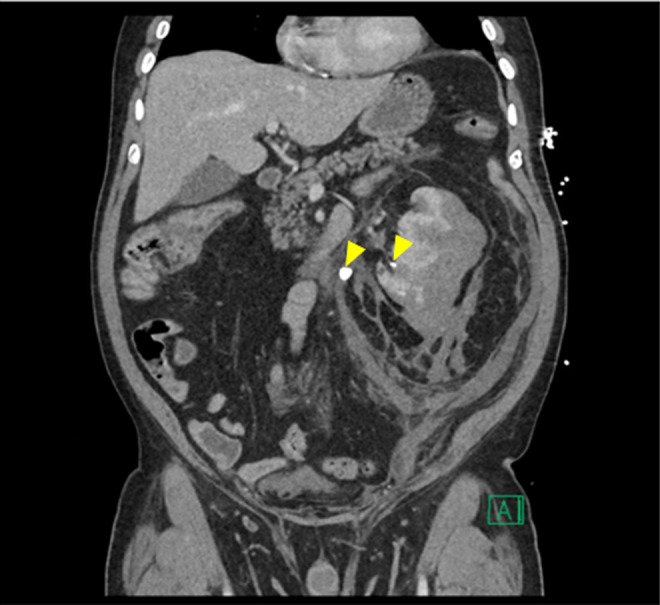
CT scan of abdomen and pelvis with IV contrast coronal section showing perinephric hematoma, nonobtructive lower pole 6 mm stone and 1.1 cm obstructive proximal ureteral stone (*arrow heads*). Marked left perinephric stranding with extension of extracapsular hematoma to distal aorta, iliac vessels, and presacral space was noted.
